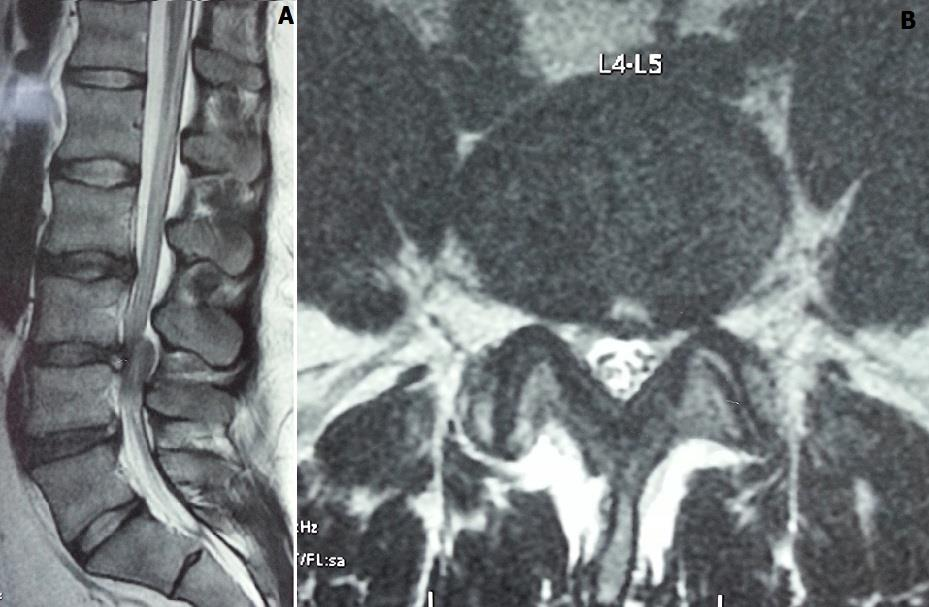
A) lumbar MRI sagittal section: mass oval shape about 2 cm height with same signal as the intervertebral disc intra ductal, extra dural seat, posterior epidural at the height of L3L4 intervertebral disk compressing the dural sheath on the same floor; B) lumbar MRI axial section: mass oval shape with same signal as the intervertebral disc intra ductal, extra dural seat, posterior epidural at the height of L3L4 intervertebral disk compressing the dural sheath on the same floor
